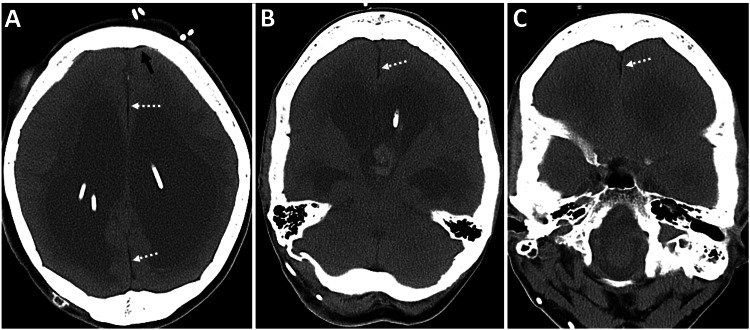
CT findings of obstructive hydrocephalus with intraventricular hemorrhage and incidental dural fat deposition in a patient with chronic shunting. A 46-year-old female presented with altered mental status, in the setting of multiple ventriculoperitoneal shunt placements for obstructive hydrocephalus. (A-C) Unenhanced axial CT images of the brain show marked supratentorial hydrocephalus with ventricular shunts and external ventricular drain catheters, along with intraventricular hemorrhage in the third ventricle and occipital horn of the left lateral ventricle. There is periventricular hypodensity, related to the transependymal flow of CSF. Incidentally, there is mild diffuse fat density along the dura in the interhemispheric falx cerebri (dashed white arrows in A-C) and along the cerebral convexity (solid black arrow in A), resulting from fatty metaplasia of the dura in the setting of chronic shunting. There is associated calvarial hyperostosis from chronic venous shunting. CT, computed tomography; CSF, cerebrospinal fluid
